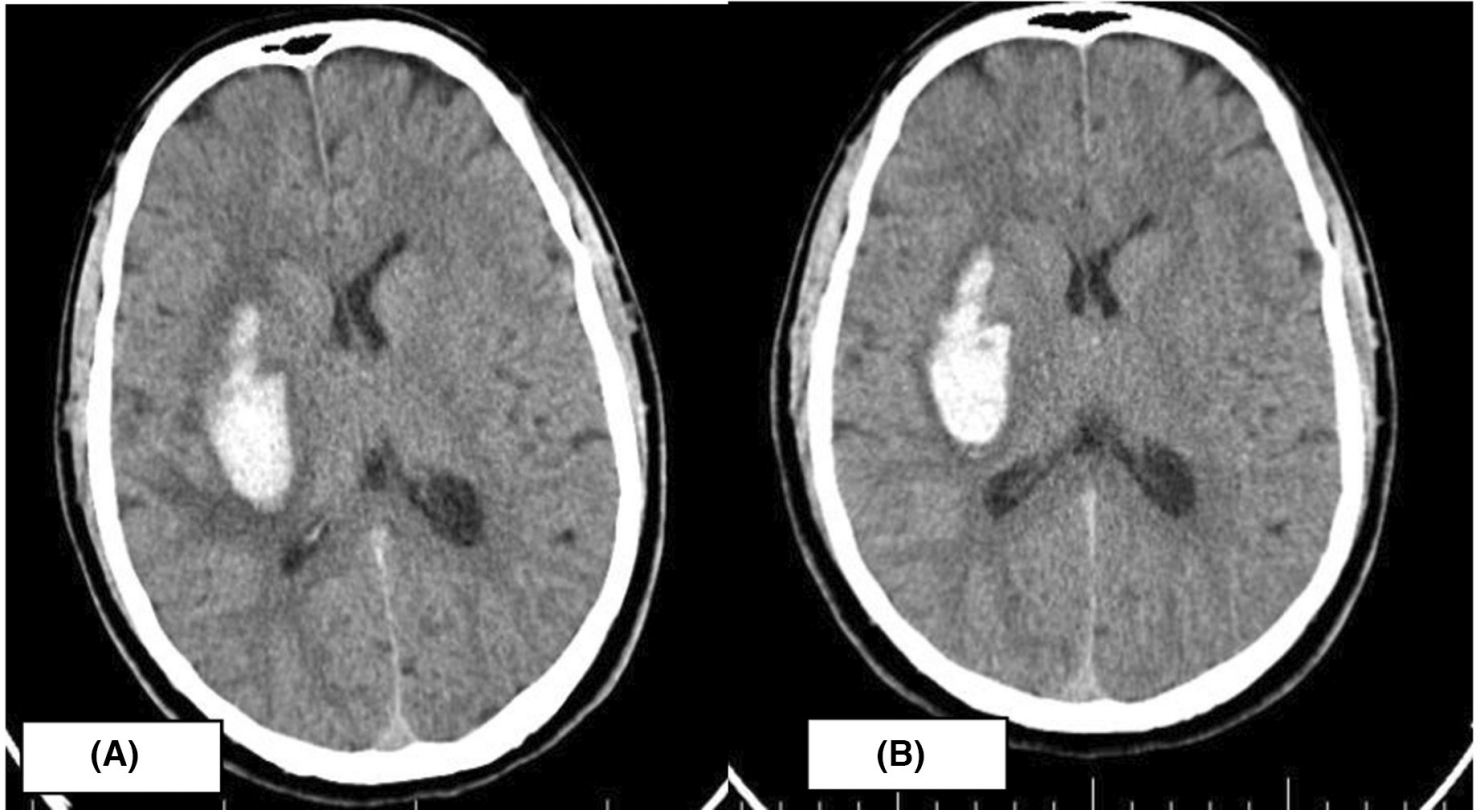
CT head showing hematoma of 53 × 24 mm in the right basal ganglia.
